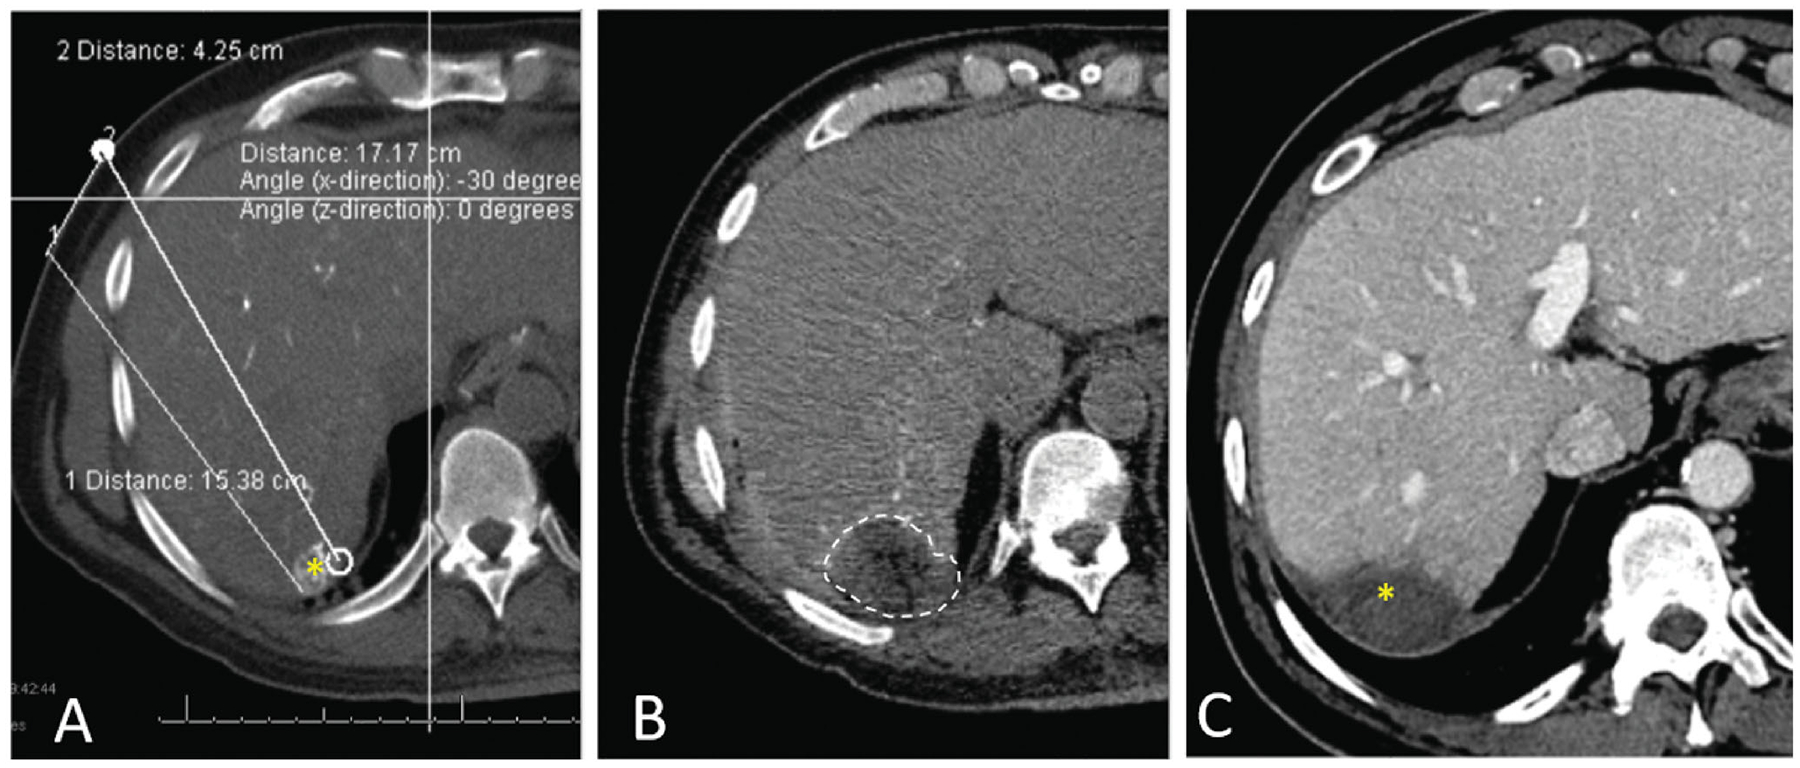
CT during hepatic arteriography (CTHA) on a patient with colorectal liver metastasis undergoing liver ablation. (A) CTHA prior to MWA antenna placement to localize target tumor (*); (B) postablation CTHA demonstrating large ablation zone (dotted line) around the treated tumor, which is no longer visualized; (C) 32-months CT follow-up demonstrating involution of the ablation zone without recurrence (*).
